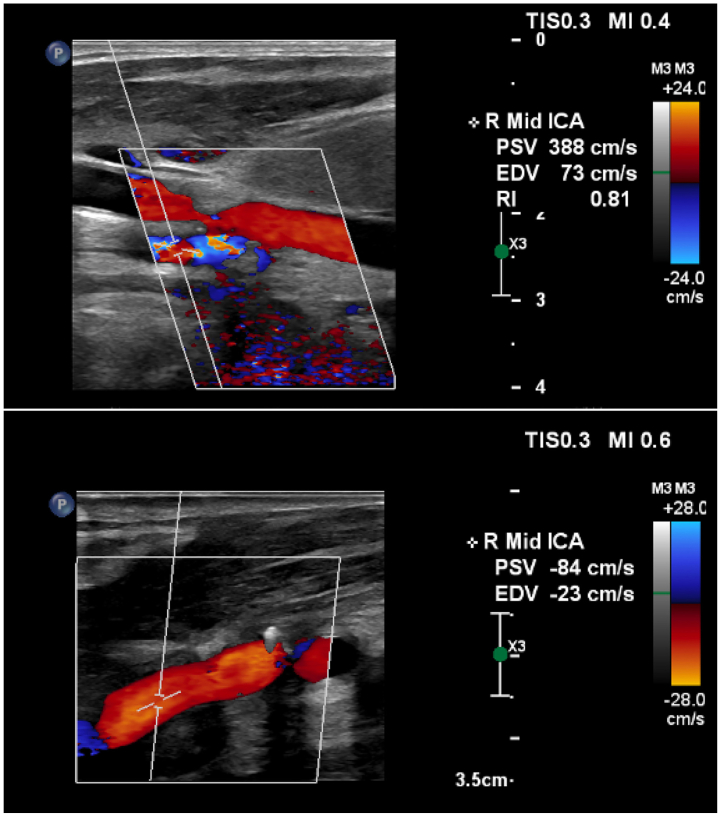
Carotid arterial duplex ultrasonography. Preoperative (*top*) right carotid artery duplex ultrasonography demonstrated preoperative flow velocities consistent with severe stenosis; follow-up imaging performed 1 month postoperatively demonstrated a widely patent right internal carotid artery (*ICA*) with no appreciable stenosis or atherosclerotic burden.
